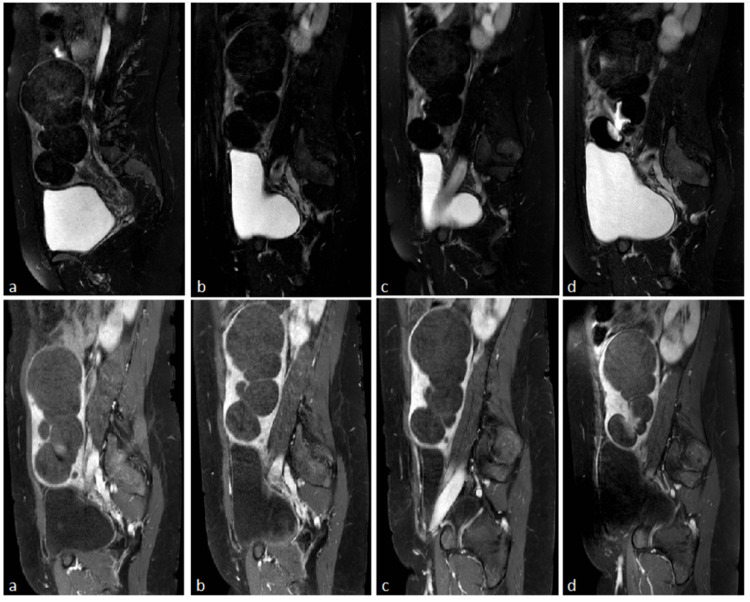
Postprocedural MRI’s Sagittal T2-weighted fat-saturated (up) and T1-weighted, contrast-enhanced, fat-saturated (down) images. a) two months follow-up, confirming significant uterus size reduction (maximum diameter 197 mm) and no enhancement with the contrast agent. b) five months follow-up, with a further reduction to the uterus size (maximum diameter 191 mm) and no enhancement of the fibroids. c) 12 months follow-up, indicating no further uterine size reduction (maximum diameter 190 mm) and unenhanced fibroids. d) 36 months follow-up, with all fibroids reappearing ischemic and in the same size as previously measured. Two submucosal fibroids at the anterior and posterior uterine walls (mirror-like) contained branching tissue infiltrations, displaying normal endometrial tissue.
